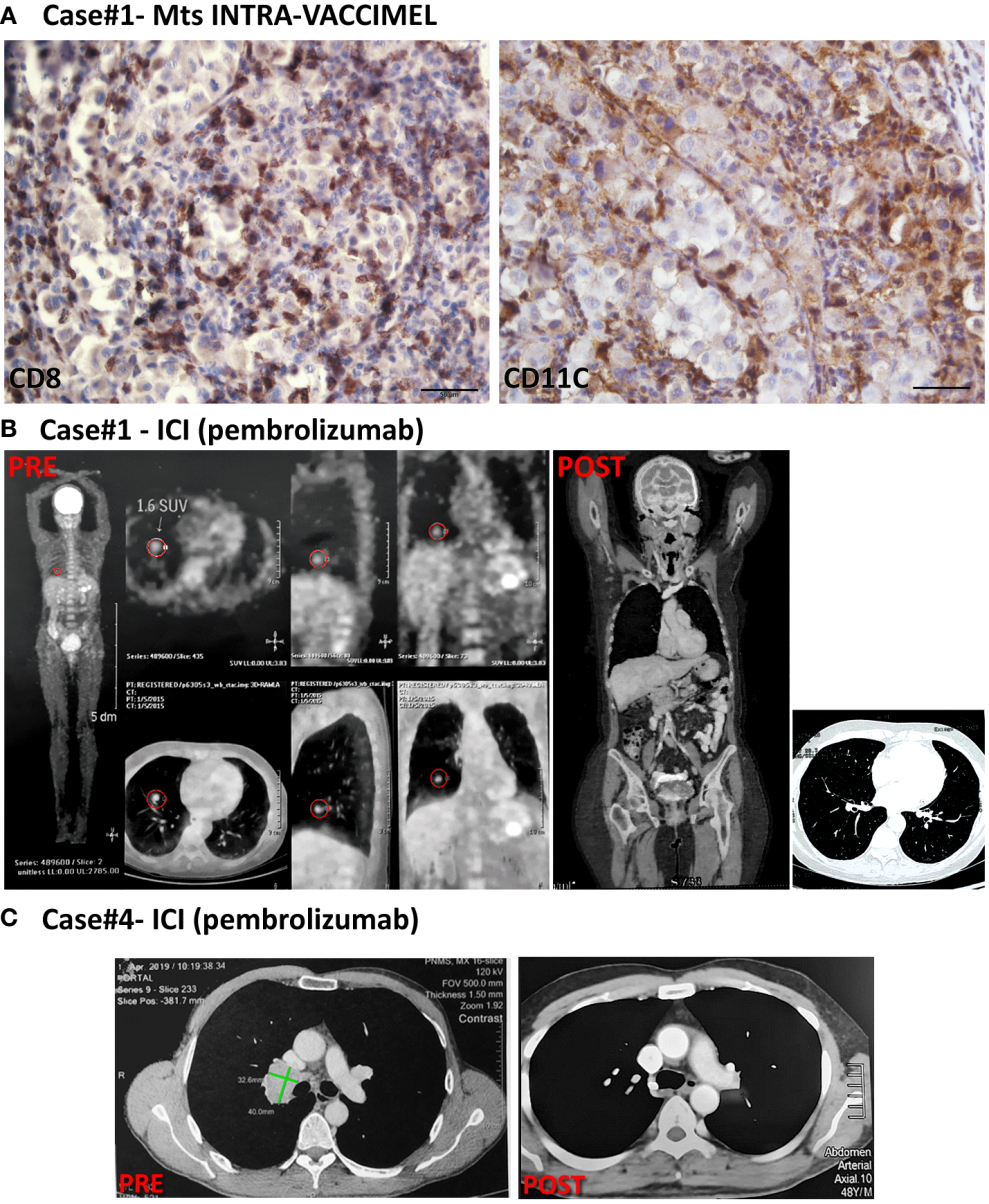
Patient #1 and #4 cases. (A) IHC of an inguinal LN metastasis excised from case#1 intra-VACCIMEL treatment; CD8^+^ and CD11c^+^ populations are shown. IHC was performed as previously described (9). Original magnification 400X; scale bars 50µm. (B) PET/CAT scan from case#1 (PRE, 01/2015) showed hypercaptating right lung nodules and a parasplenic nodule. After pembrolizumab treatment, this patient attained complete remission in 04/2018 (POST) and remained NED until present (09/2023). (C) CT from case#4, dated 04/2019 (PRE) showing lung right lung hilar metastases– (indicated by green lines), not detectable after treatment with Pembrolizumab (POST; dated 07/2020).
